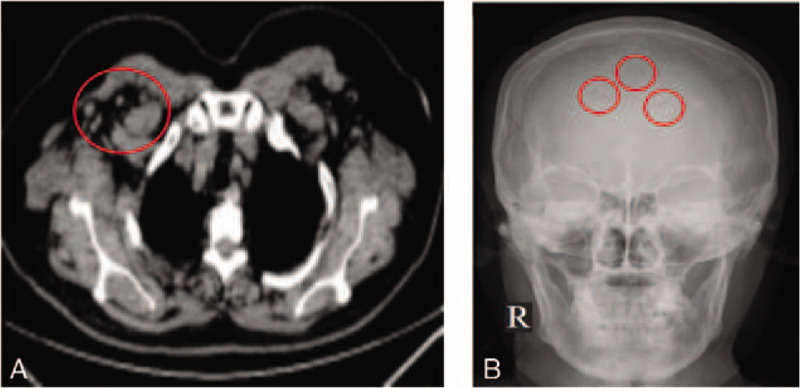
Thorax computed tomography and X-ray of skull.
